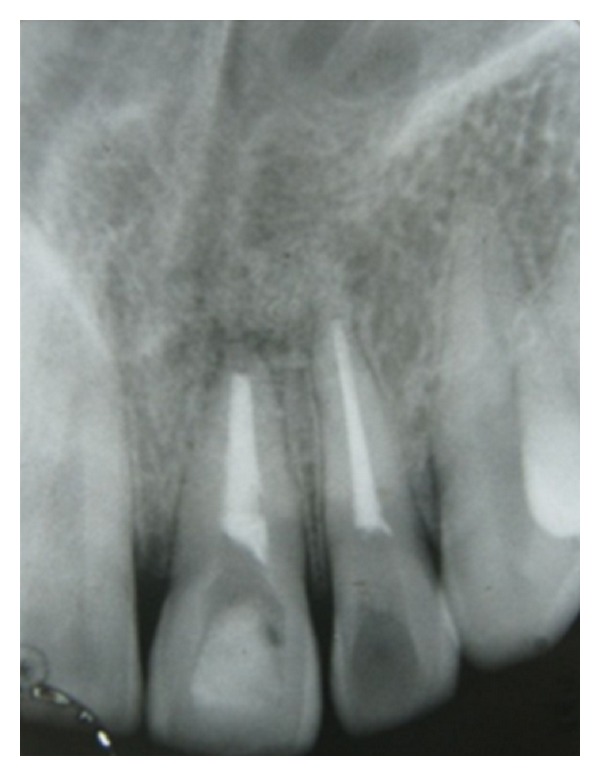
Follow-up radiograph after 6 months (with intracoronal bleaching agent).
